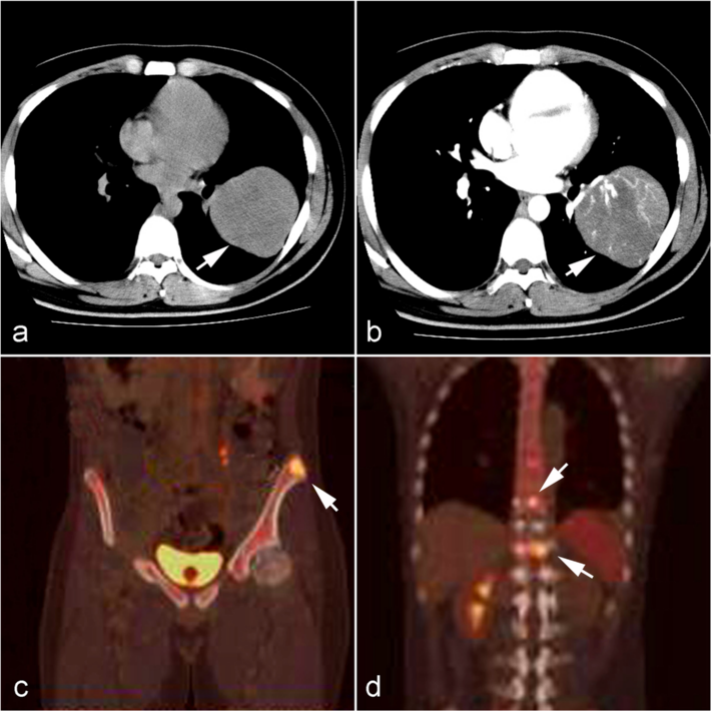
Radiological findings of lung tumor and metastatic lesions of bone. a Chest computed tomography (CT) revealed a huge well-circumscribed mass in the left lower lobe of lung without signs of neighboring pleura invasion (*white arrow*). b The pulmonary tumor showed heterogeneous enhancement. Three months after surgery, F18-fluorodeoxyglucose (FDG) positron emission tomography revealed abnormally increased FDG uptake in the pelvic bone (c) and vertebra mass (d) (*white arrows*), indicating metastatic lesions of bones
